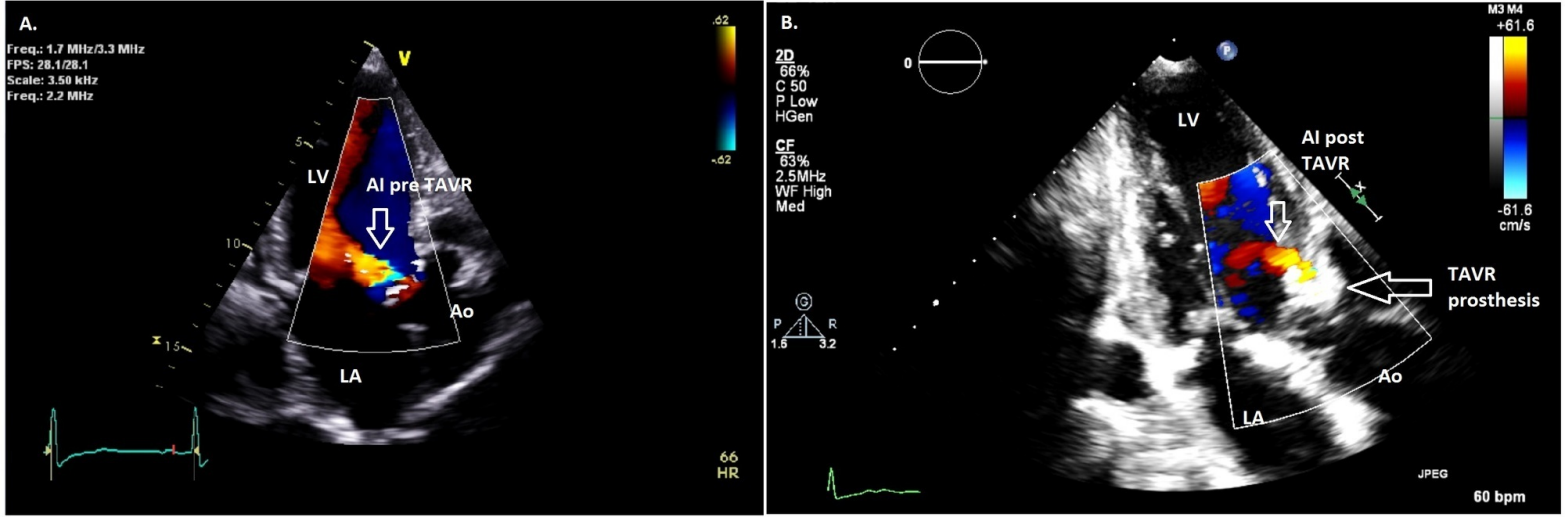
Improvement in severity of aortic insufficiency on Doppler echocardiography - before (A) and after transcatheter aortic valve replacement (TAVR) (B).
